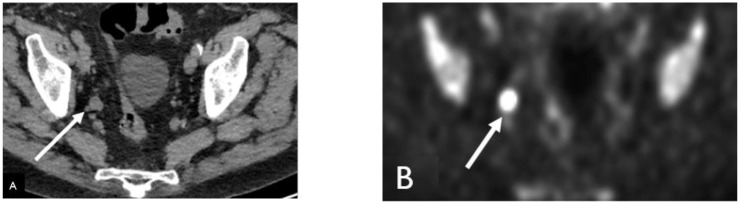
Sixty-nine-year-old male with history of prostate cancer, status post-prostatectomy and salvage radiation, now with rapidly rising PSA. Non-contrast-enhanced-CT (NECT), (A) shows an enlarged right pelvic side wall lymph node. Corresponding Fluorine-18 Fluciclovine (Axumin) PET image (B) shows intense radiotracer uptake concerning for recurrence and nodal disease.
